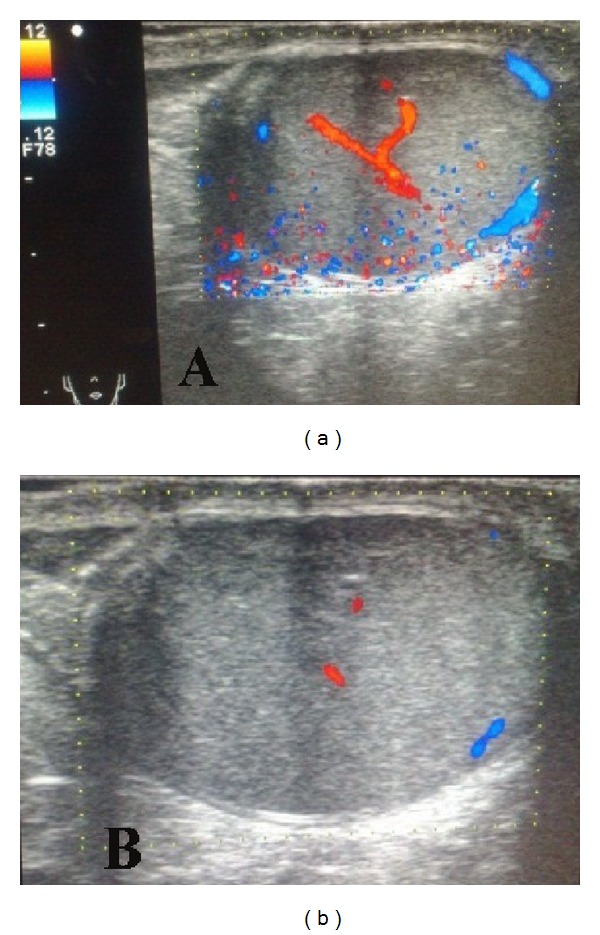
(a) Testicular Doppler U/S showed no signs of ischemia with good blood flow. (b) Testicular Doppler U/S showed poor blood flow.
